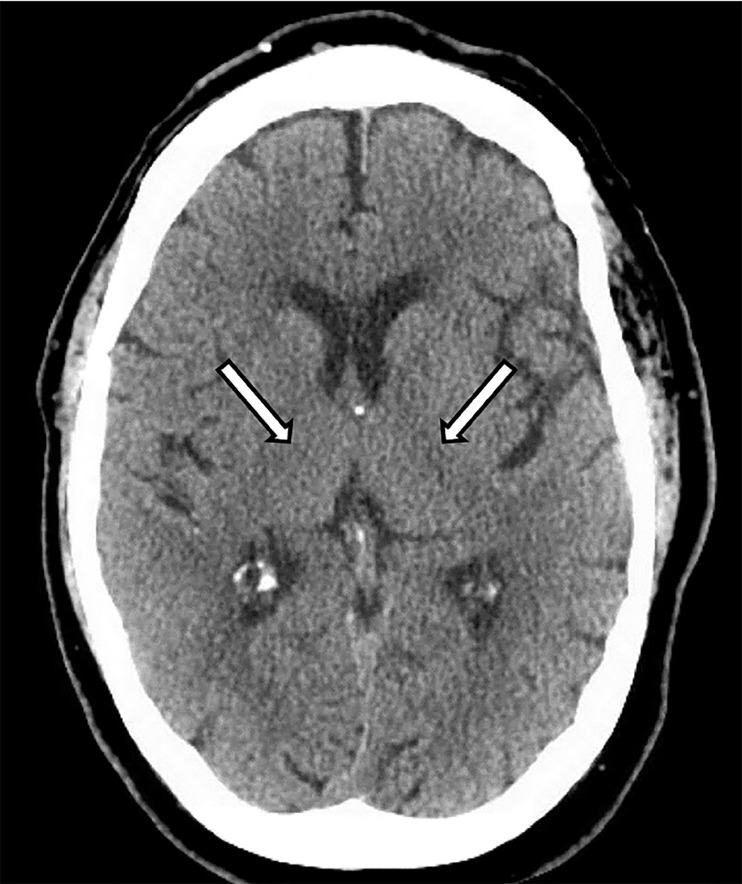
Computed tomography (CT) brain of patient with Wernicke encephalopathy (WE). The utility of CT in WE is primary to rule out alternative diagnoses. Here, there is periventricular hypoattenuation of the white matter (arrows) without evidence of any other alternative diagnoses. This is nonspecific but consistent with chronic, small-vessel ischemic disease, which is consistent with his baseline mild vascular dementia but is insufficient as an explanation for his more acute neurological deficits. Magnetic resonance imaging would demonstrate findings specific for WE. Notably, this altered patient had difficulty remaining still for the examination, giving rise to significant motion artifact.
